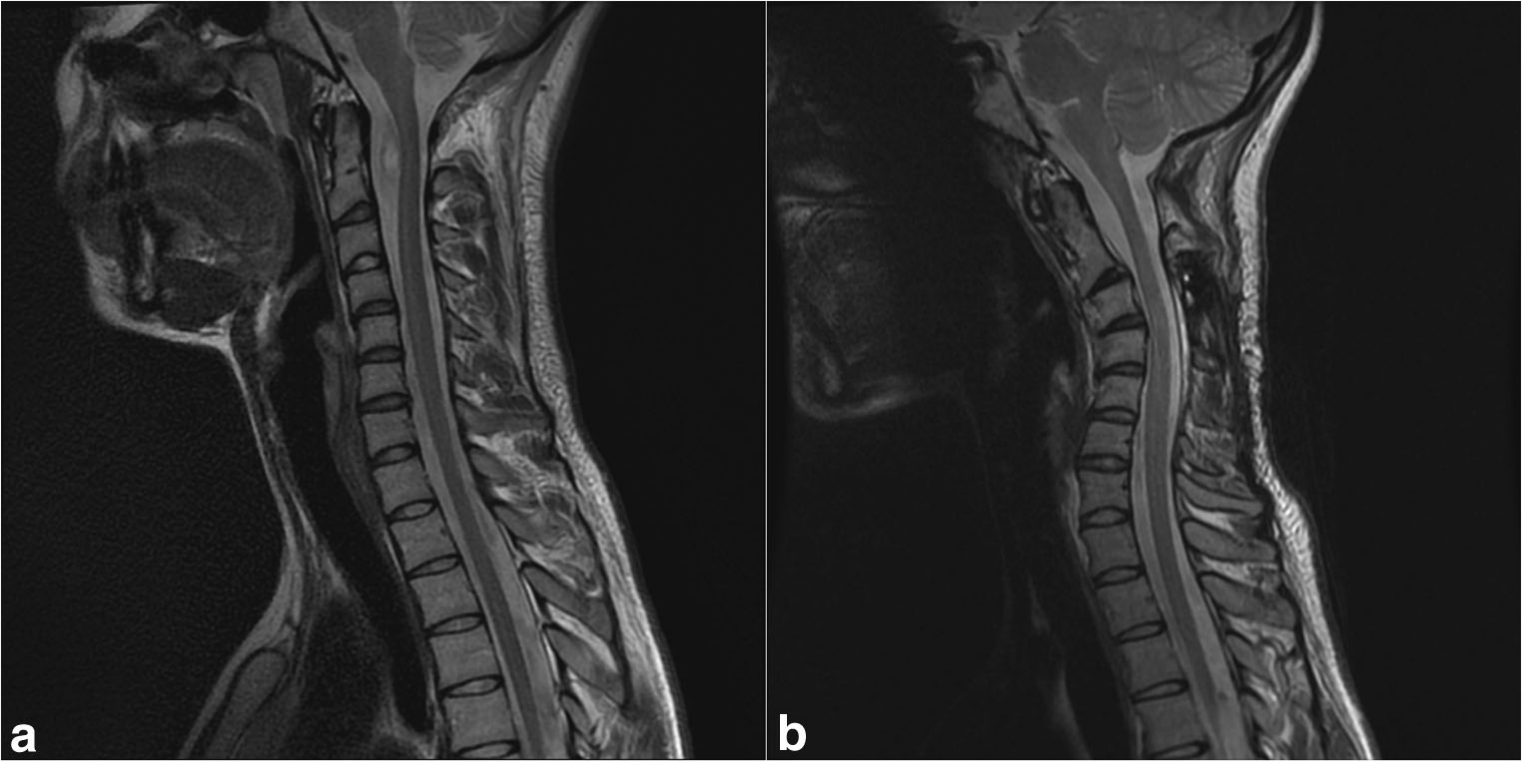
Pre- (a) and postoperative (b) magnetic resonance image showing kyphosis following cervical laminectomy
